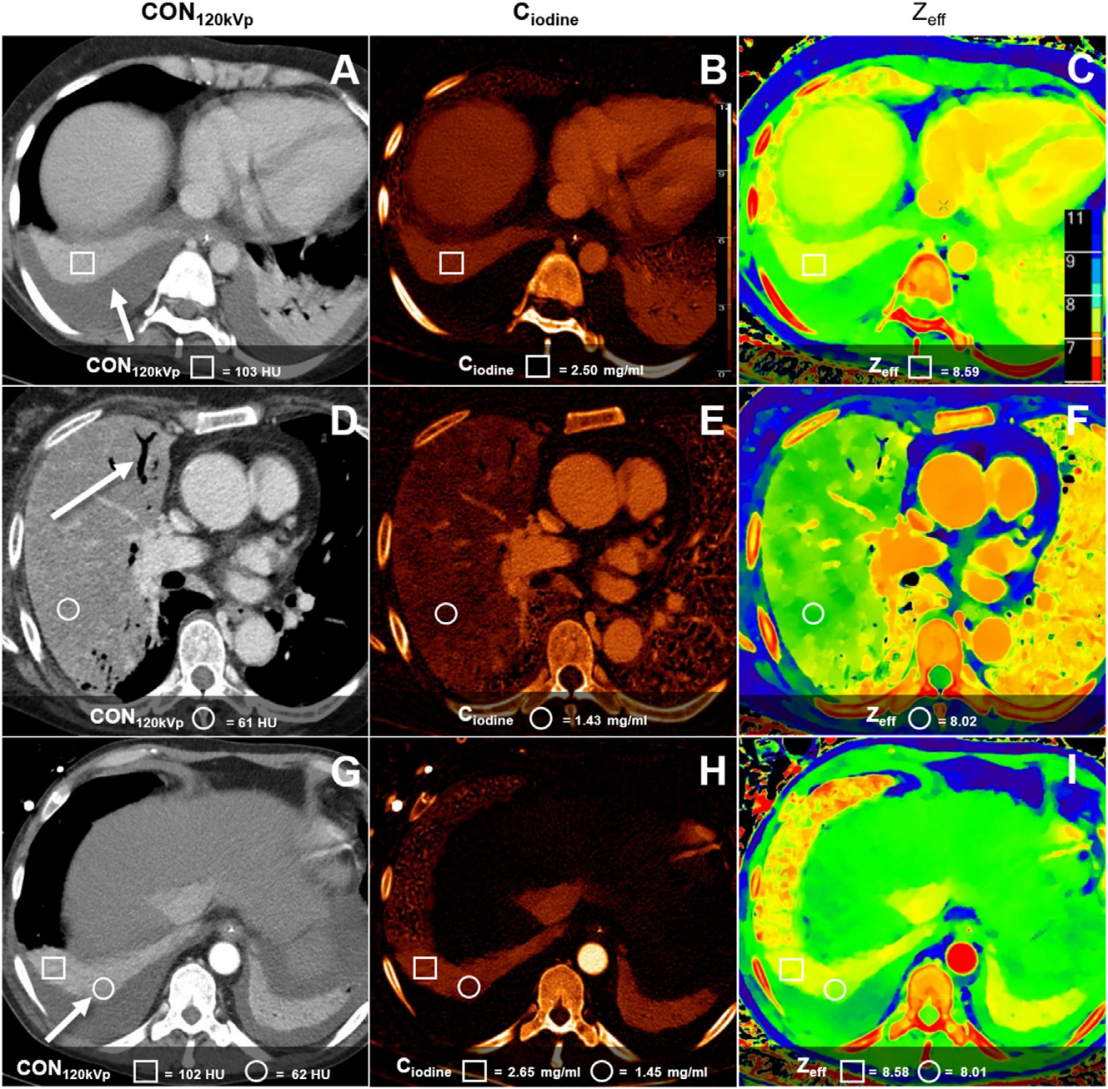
Conventional, iodine concentration, and effective atomic number images of contrast-enhanced chest CT in three different patients. (A, B, C) Bilateral pleura effusion within adjacent homogenous atelectasis (white arrow) in a 45-year-old woman. (D, E, F) Right-sided lobar pneumonia with a positive air bronchogram a 51-year-old woman. (G, H, I) Bilateral pleural effusion with adjacent atelectasis in a 45-year-old man. On the right side is a focal area with hypoperfusion within the atelectasis (white arrow), which is suspicious for a superinfection of the atelectasis. Quantitative CT parameters are shown for atelectasis (square) and pneumonia (circle).
